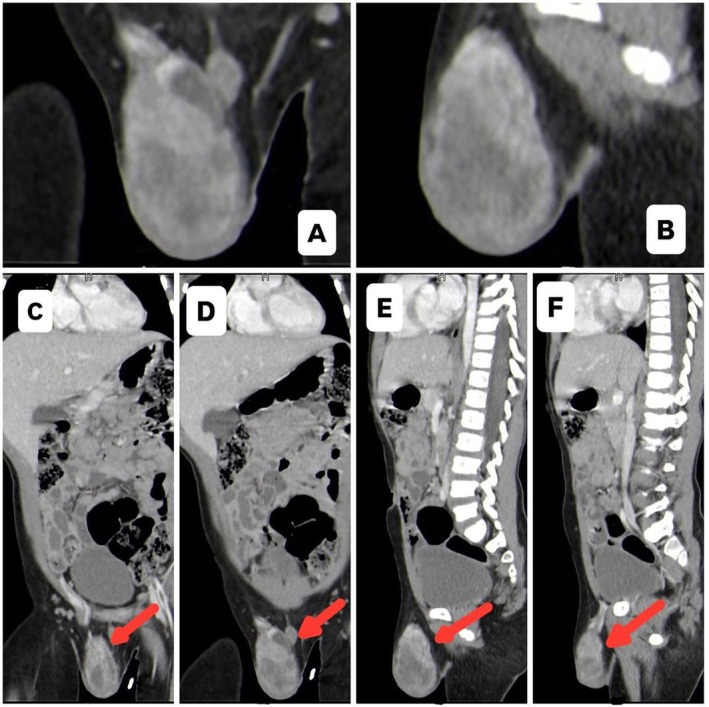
(A, B) Zoomed axial images better depict the morphology and extent of the right scrotal mass. (C, D) Coronal CT cuts demonstrate the cranio‐caudal extent of the lesion along the spermatic cord with no other distant visceral metastasis. (E, F) Sagittal CT cuts show the relationship of the mass with the surrounding scrotal structures and confirm the absence of overt local invasion.
